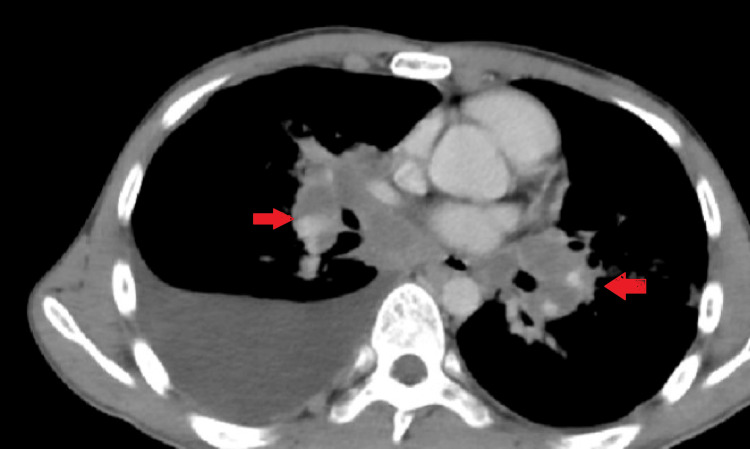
Mediastinal window of CECT thorax showing bilateral hilar lymphadenopathy (red arrows). CECT: contrast-enhanced computed tomography
